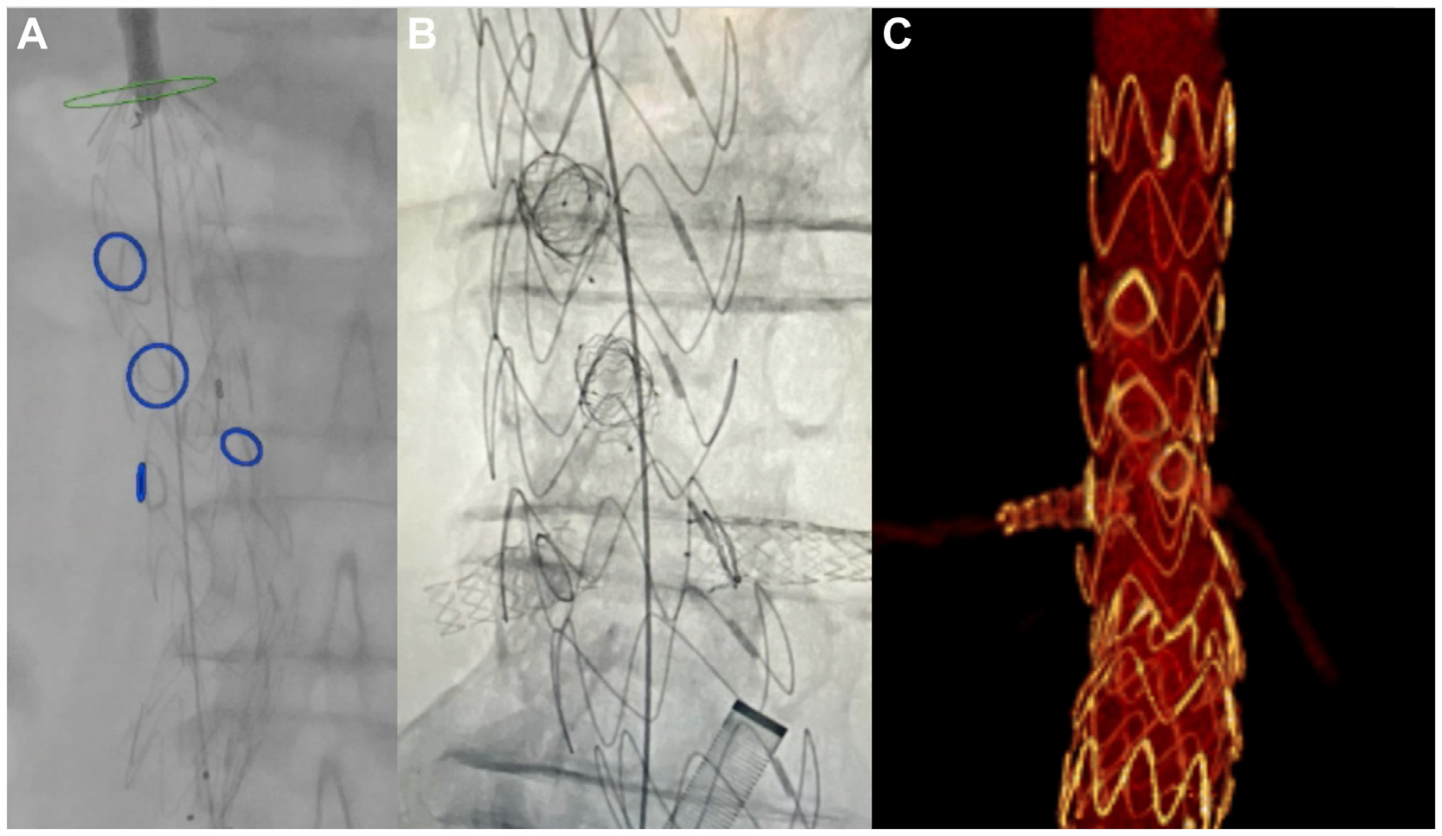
A, Intraoperative image showing physician-modified endograft (PMEG) deployment. Note the alignment between the fenestrations and overlay fusion rings indicating the origin of the target vessels. B, Intraoperative fluoroscopy image after PMEG deployment and positioning of the bridging stents. C, Three-dimensional image of the postoperative computed tomography angiogram.
